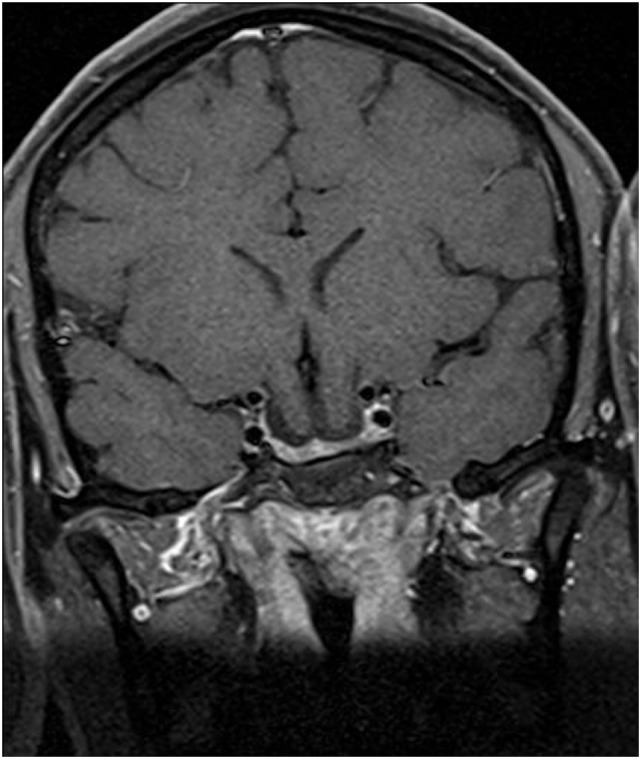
Coronal postcontrast T1-weighted image 5 months after therapy with bromocriptine.
